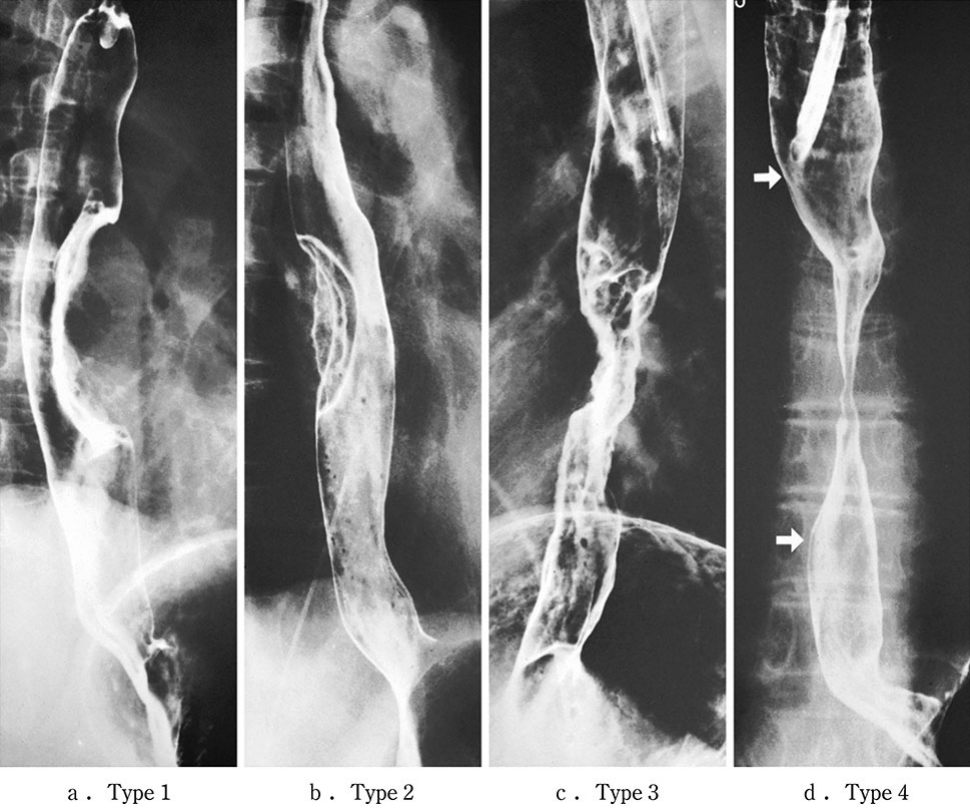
Roentgenological findings: advanced type
